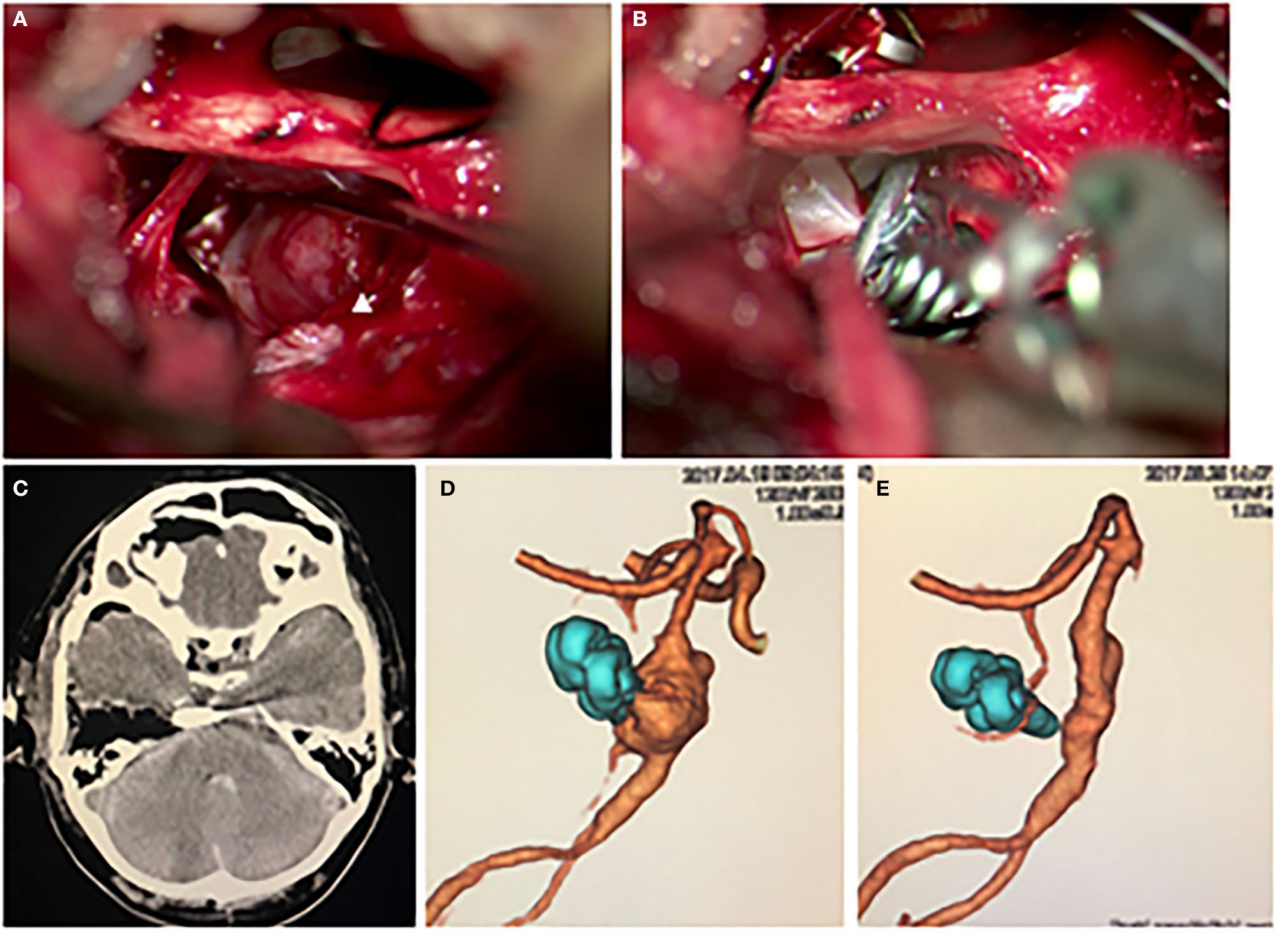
(A,B) The intraoperative findings. (A) The aneurysm was observed via the anterior transpetrosal approach. The white arrowhead indicates a bleb on the right shoulder of the aneurysm, which was covered by hematoma, indicating the bleb was the rupture point. (B) The aneurysm, including the bleb, was partially occluded with a combination clip using three clips. The photograph shows the third clip is being applied. (C) Postoperative CT showed no complication. (D) Postoperative 3D CT angiography showed the disappearance of the bleb. (E) A remarkable regression of the aneurysm was observed on 3D CT angiography 1 year after the surgery.
